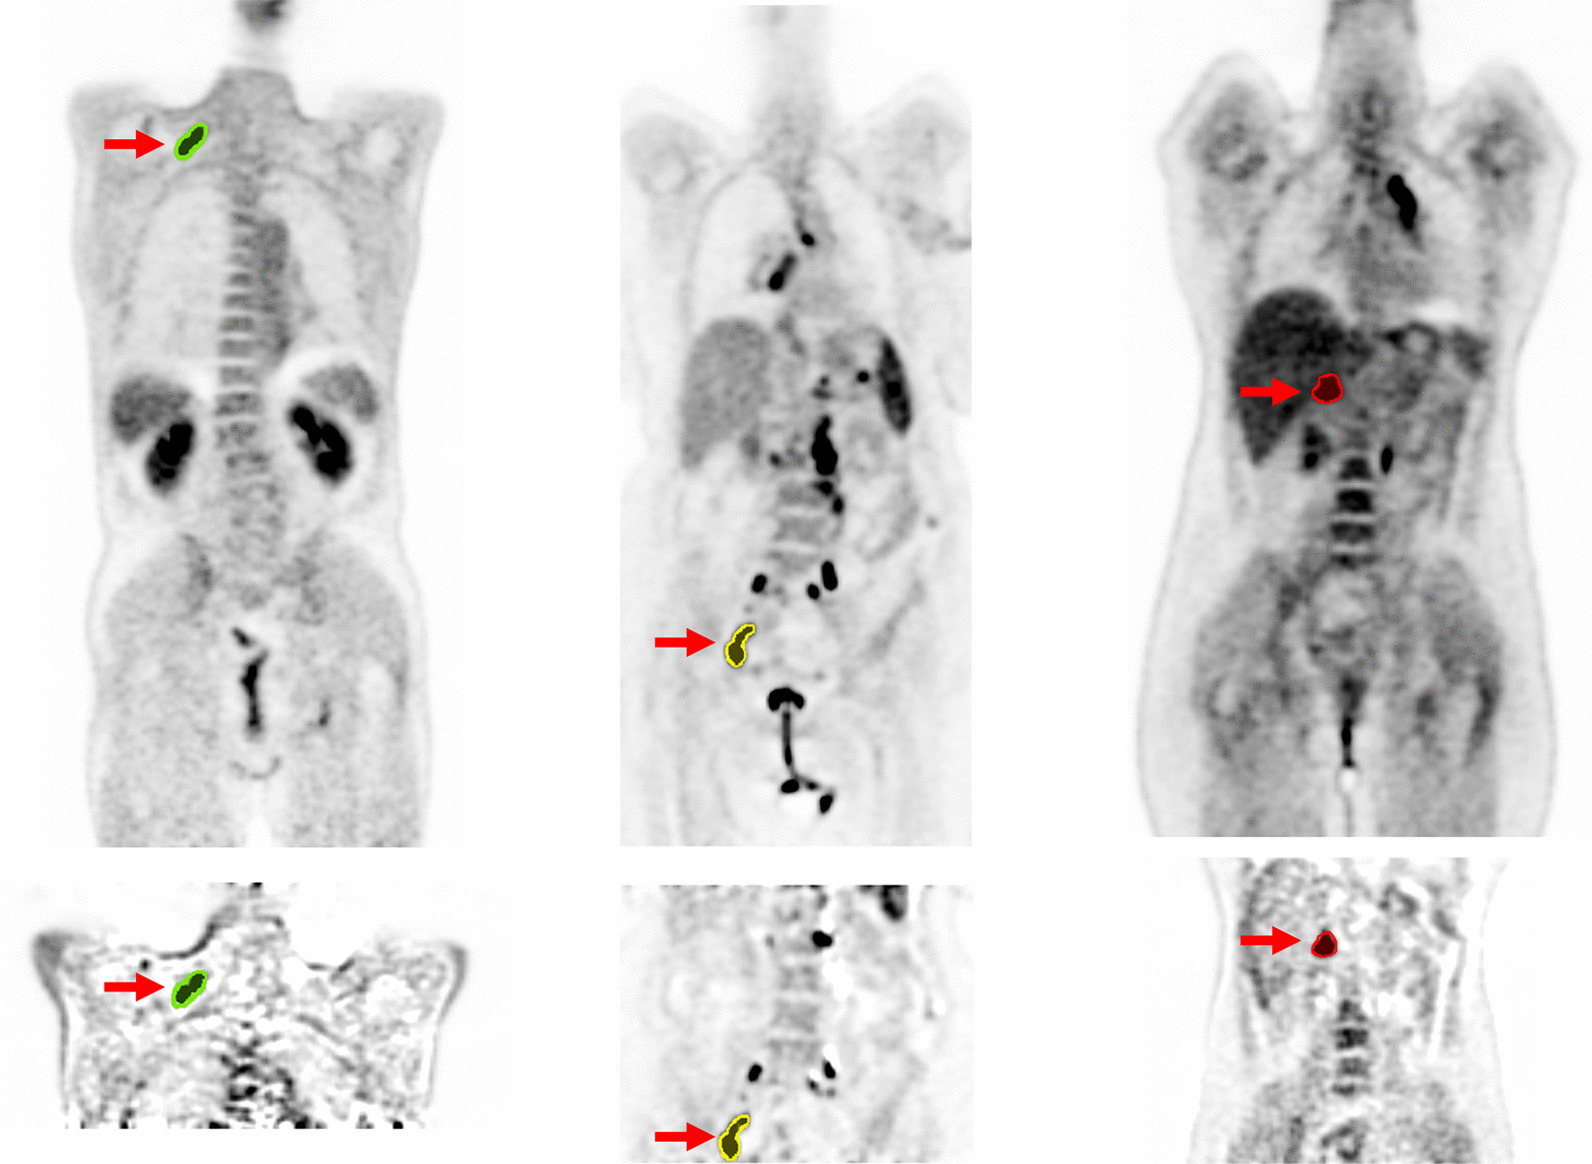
Examples of SUV (top) corresponding the DTP K_i_ (bottom) images showing segmented lesions
